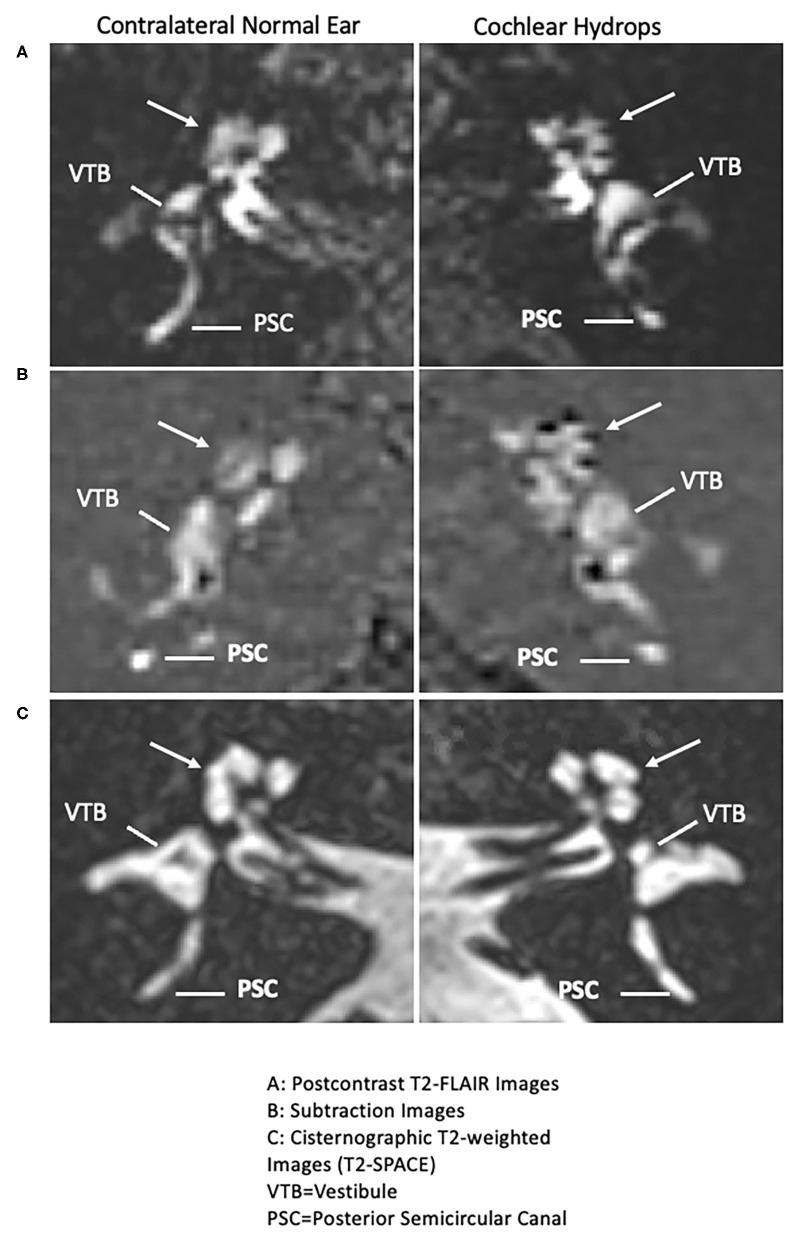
Isolated cochlear endolymphatic hydrops on 3T MRI: *Right* column: Cochlear hydrops and *Left* column: contralateral normal ear. (A) (Top right panel: Cochlear hydrops), delayed postcontrast T2-FLAIR images through the cochlea demonstrate a prominent scala media signal void consistent with a dilated cochlear duct (arrow) in the left ear (Top left panel: normal contralateral side), a normal appearing cochlea in the right ear (arrow). Posterior semicircular canal (PSC) and vestibule (VTB) are labeled for reference. (B) (Middle right panel: cochlear hydrops), corresponding subtracted images more clearly isolate the cochlear duct (arrow) delineated against a nullified background (Middle left panel: normal contralateral side), a normal appearing cochlea in the right ear (arrow). (C) Bottom right panel: Cochlear hydrops and Bottom left panel: normal contralateral side): both sides on corresponding cisternographic T2-weighted images (T2 SPACE), provided for anatomic reference, demonstrate normal fluid signal within the vestibule and cochlea on the disease side and the normal contralateral side, indicating isolated cochlear hydrops.
